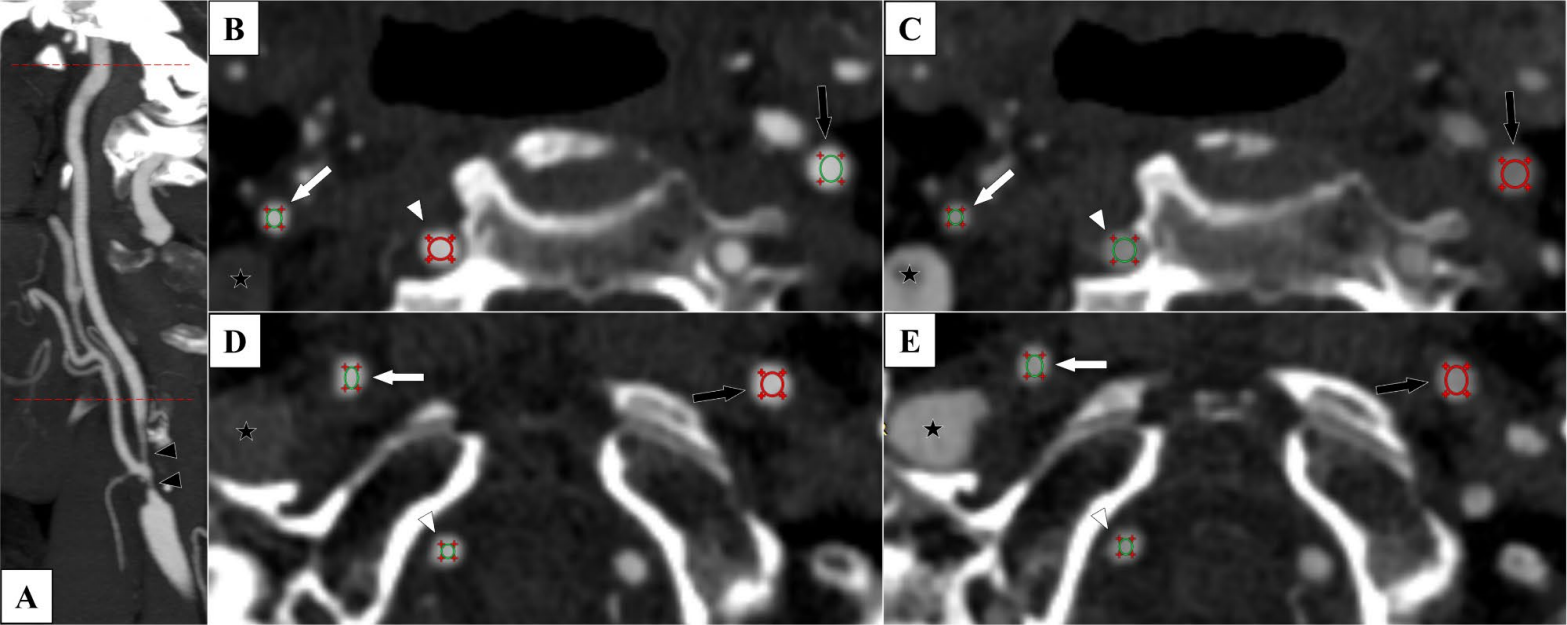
The 12 measurement points in biphasic CTA exams. Case with right-sided near-occlusion without full collapse. 7 s between arterial and venous phase. ROIs placed to avoid the fuzzy edge around the artery. (A) Sagittal view with cervical levels (proximal and distal) marked with red dotted lines. Note that the proximal measurement still refers to the artery beyond (distal to) the stenosis (black arrowheads). (B) Arterial phase, proximal level. 488, 542 and 535 HU in right ICA (white arrow), left ICA (black arrow) and vertebral (white arrowhead). (C) Venous phase, proximal level. 351, 274 and 332 HU. Note the filling of the right jugular vein (black star with white rim). (D) Distal level, arterial phase. 486, 521 and 477 HU. Note the non-intuitive change in vertebral HU between proximal and distal phase that shifts the direction of v-correction from − 47 to + 9 HU whereas c-correction is more intuitive (− 54 and − 38 HU). (E) Distal level, venous phase. 379, 343 and 386 HU.
